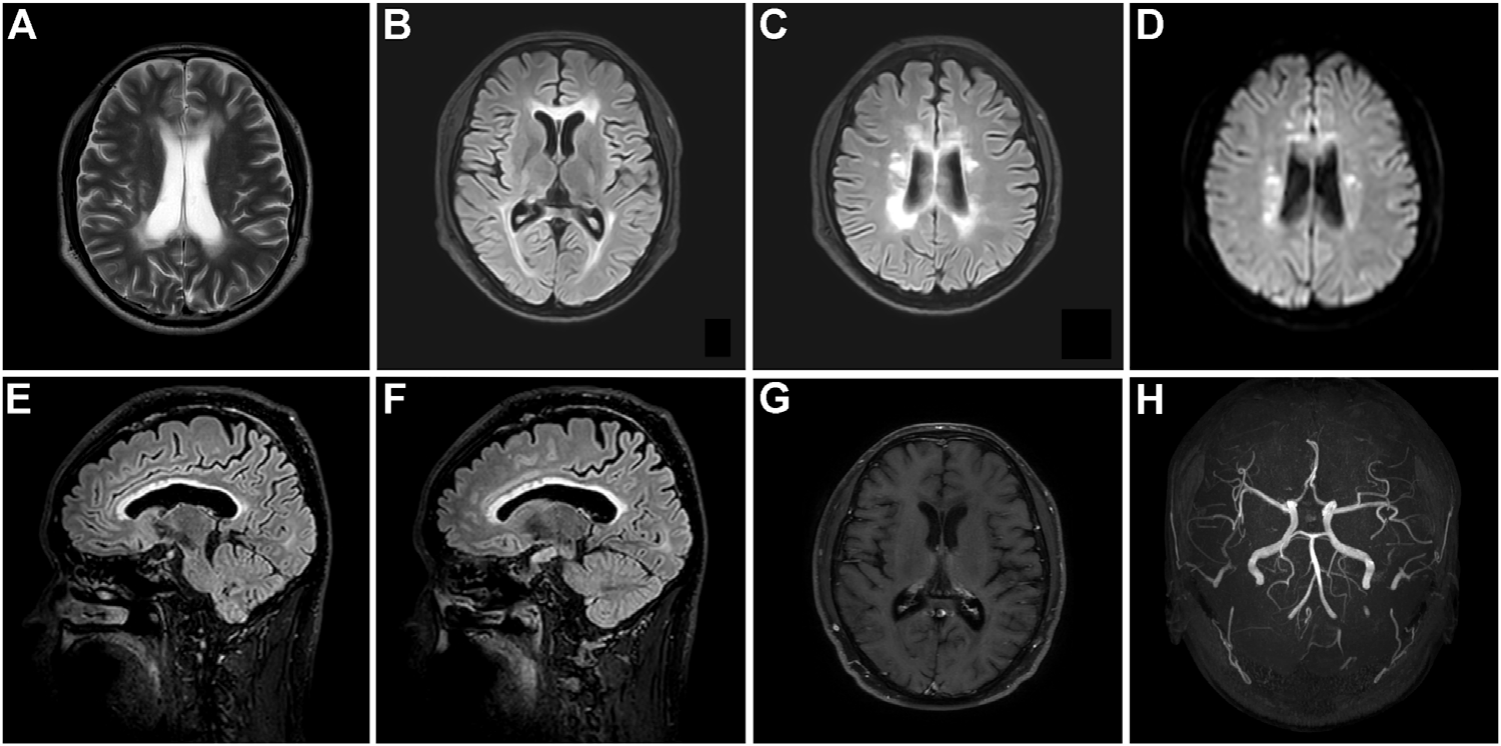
Brain MRI of the proband with CSF1R-microglial encephalopathy in the first family. Bilateral confluent and diffuse deep white matter hyperintensities involving periventricular areas, corona radiate and the corpus callosum, and moderate diffuse atrophy of the bilateral frontoparietal lobes can be seen on brain axial T2-weighted imaging (A), fluid-attenuated inversion recovery (FLAIR) (B,C) and diffusion-weighted imaging (D). Sagittal brain FLAIR shows abnormal hyperintensities and thinning of the corpus callosum, and extensive cortical atrophy (E,F). No obvious abnormalities can be found in the Gadolinium contrast-enhanced MRI (G) and cerebral arteries on magnetic resonance angiography (H).
